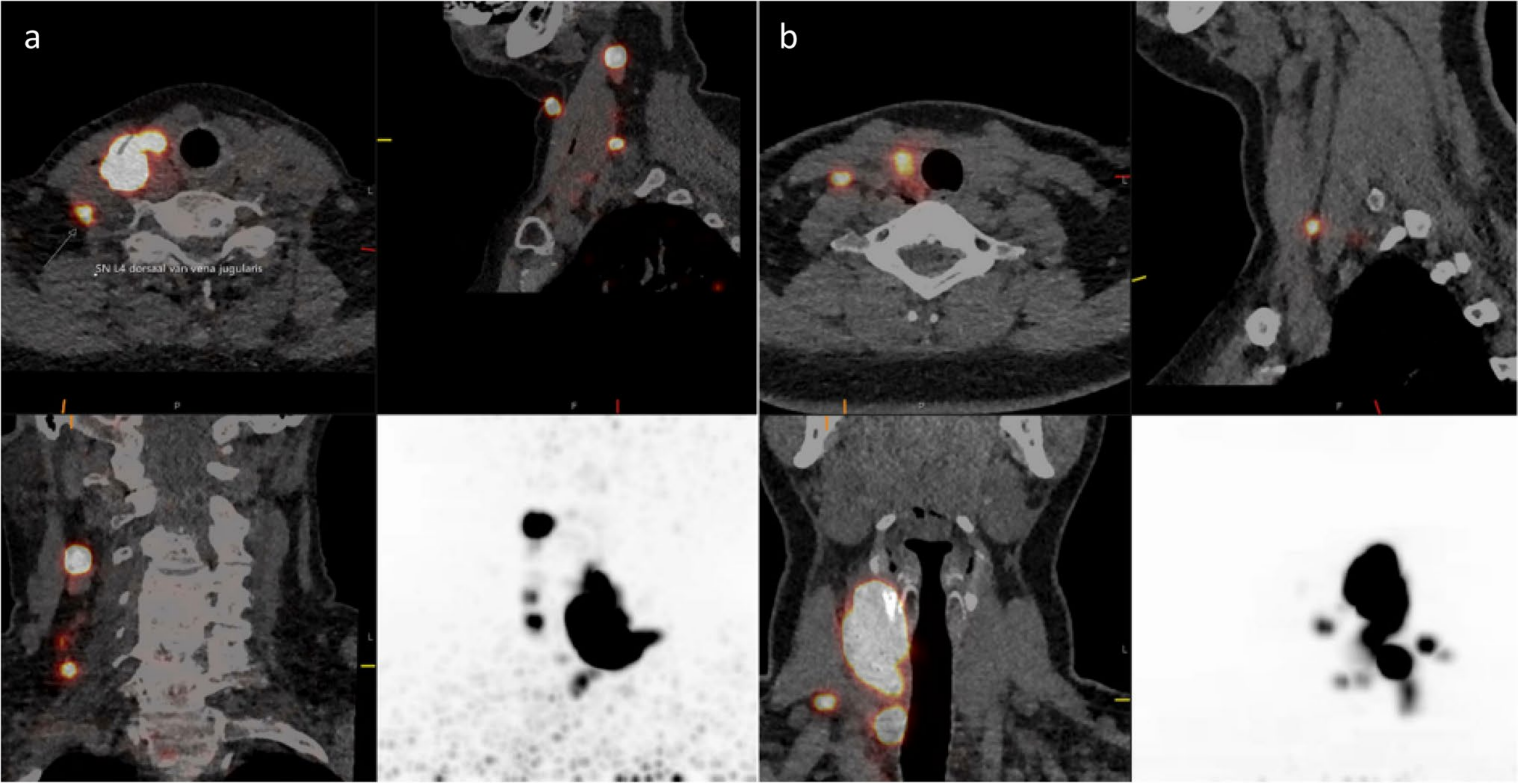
^68^Ga-tilmanocept PET/CT images of (a) patient no. 7 showing SLNs in levels 2 and 4 and aspecific paratracheal uptake not related to a lymph node and (b) patient no. 3 with SLNs in levels 3, 4, and 6
